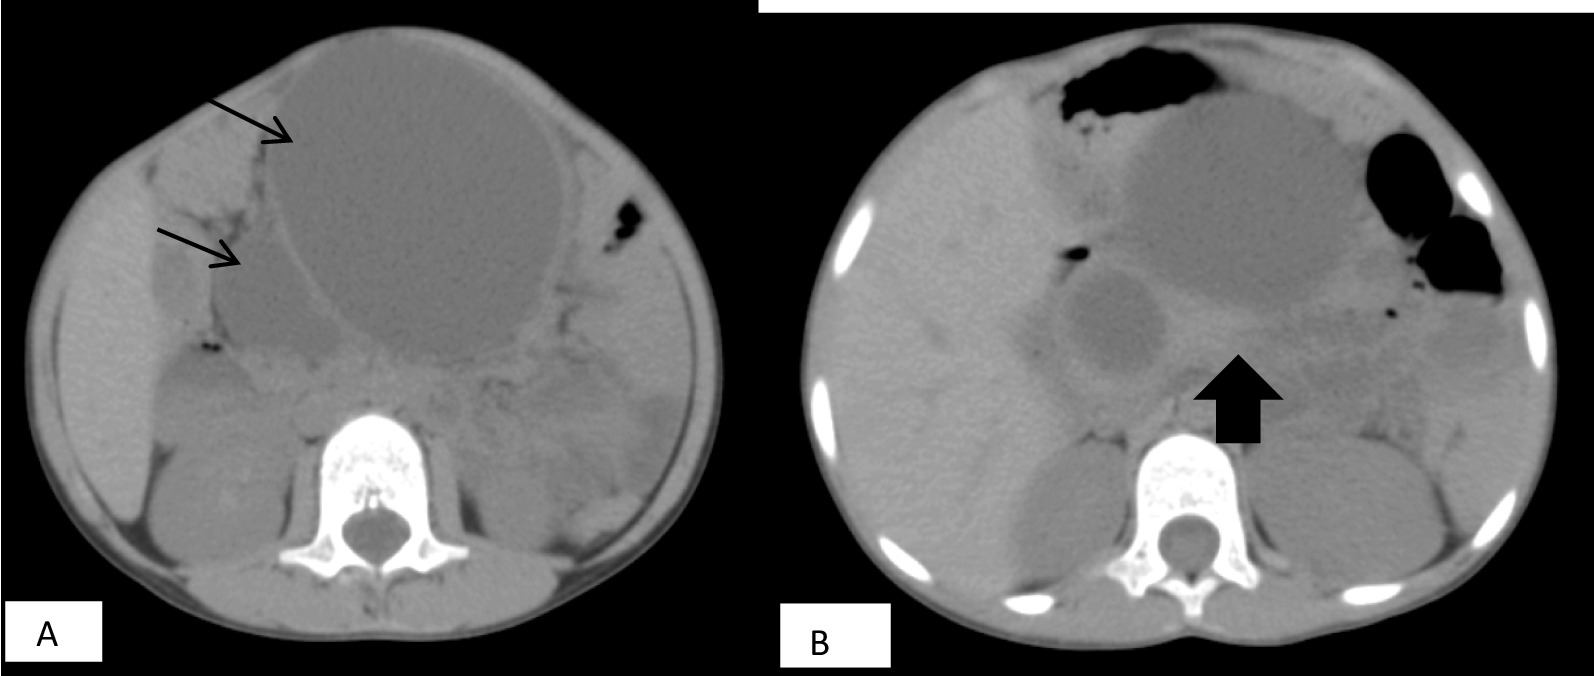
Computed tomography scan of pancreatic lymphangioma. A Axial view both upper and lower arrows showing different size non-communicating multicystic retroperitoneal mass. B As depicted by the arrow, no clear plane is seen between the mass and the pancreatic tissue
